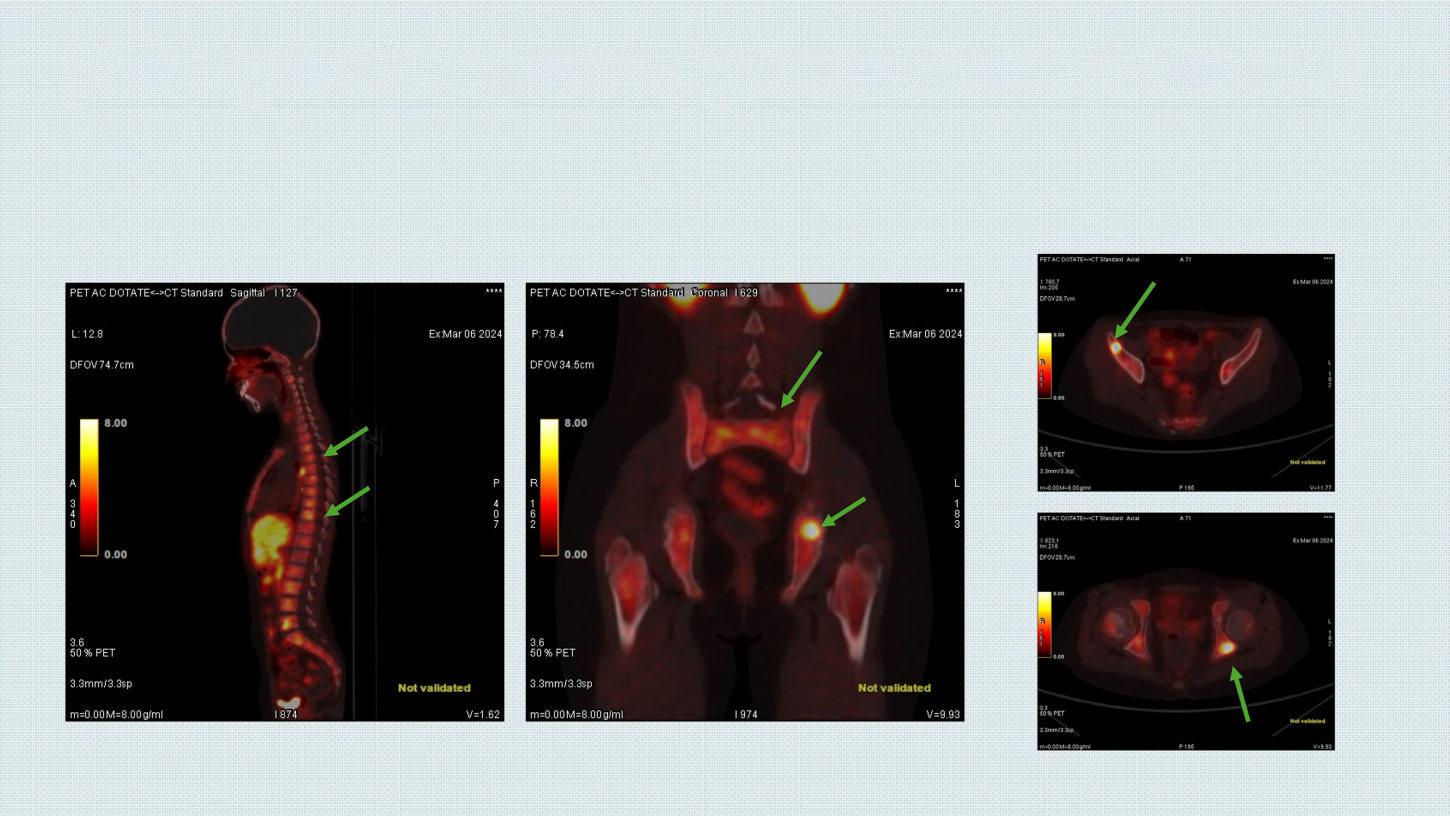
Bone involvement on DOTA-PET/CT image before combination treatment with lorlatinib and naxitamab.
